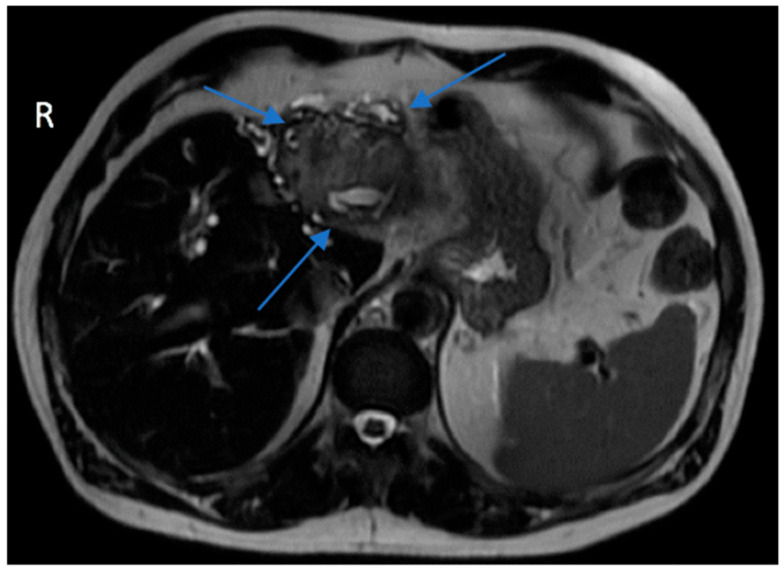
Axial view of the T1-weighted MRI abdomen MRCP with and without contrast showed an intrahepatic cholangiocarcinoma (blue arrows) consistent with the findings on the CT scan. The hypointense lesion extended from the hepatic hilus into the left lobe.
